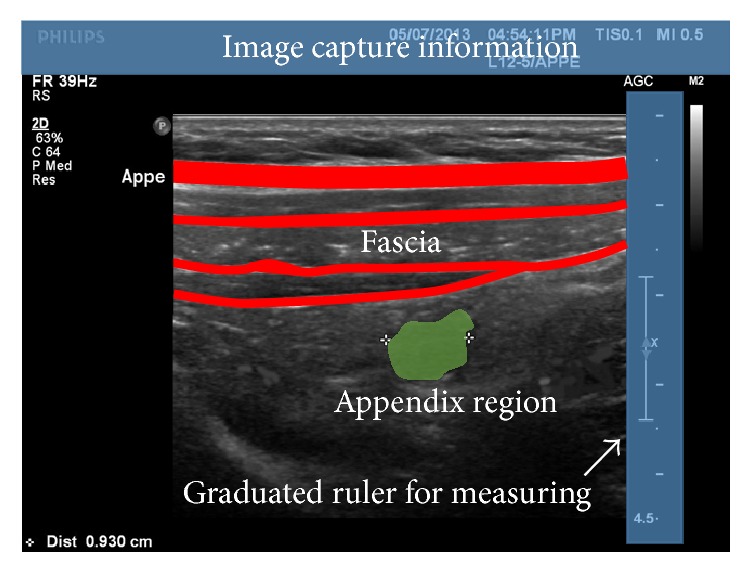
Typical input ultrasound image.
